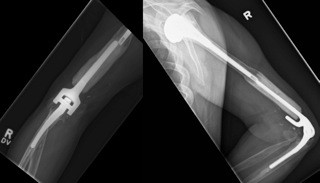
Anteroposterior and lateral radiographs of the elbow at 28 and 24 months post operation, demonstrating incorporation of the allograft into the host humerus.
